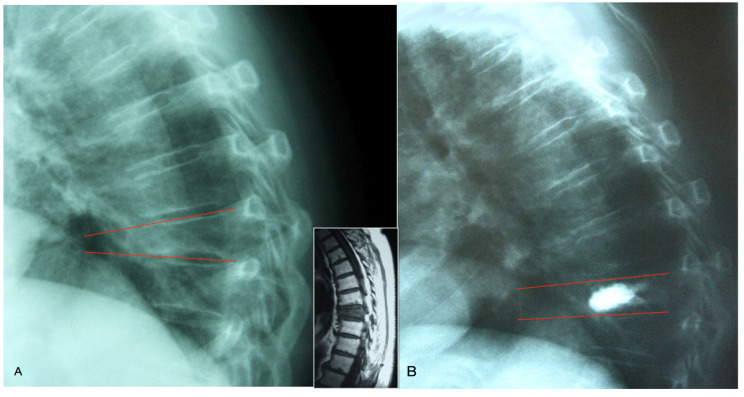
Measuring the kyphotic angle. It was calculated as measuring the angles between lines running parallel to the upper and lower endplates of fractured vertebrae before and after surgery (A,B) (Sagittal MRI showing bone edema due to acute fracture).
